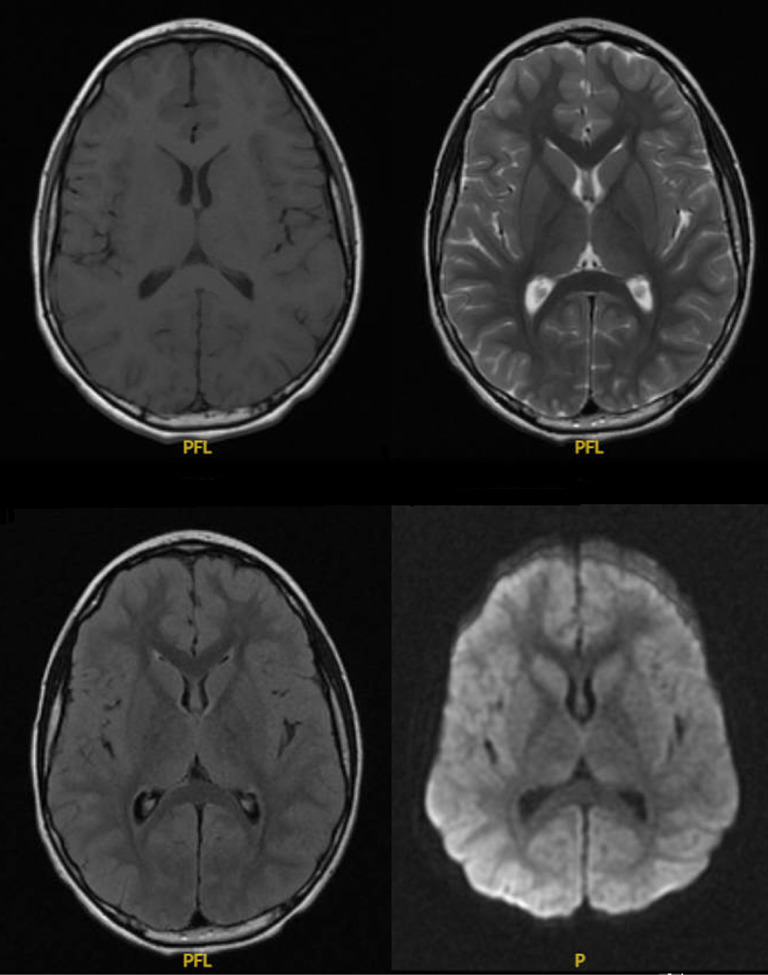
Clockwise from top left: Normal Axial T1, T2 FSE, Diffusion Weighted Imagine, and T2 FLAIR.
